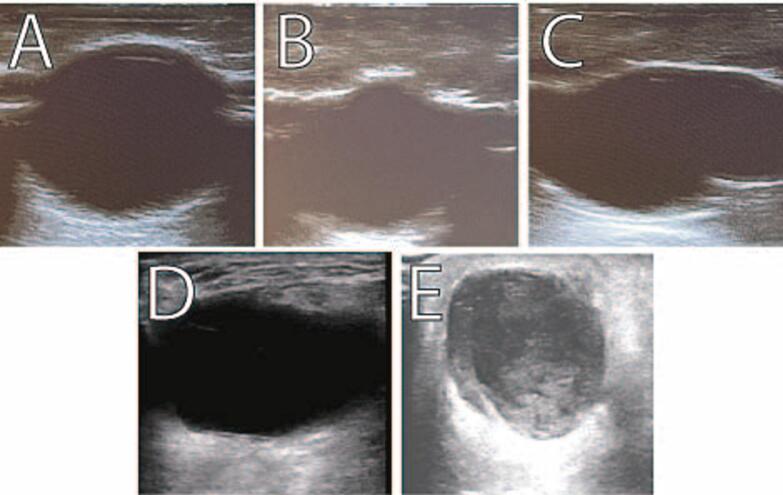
Representative examples of the abscess model (A, B, C) as seen on ultrasound. The images in D and E represent a real-life ultrasound evaluation of an abscess [10,11].
